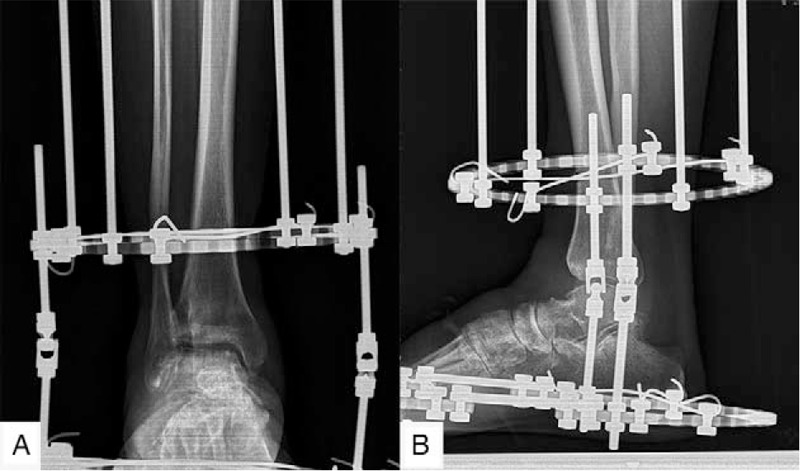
The weight-bearing radiograph 3rd month postoperative showed that the joint space remained good.
